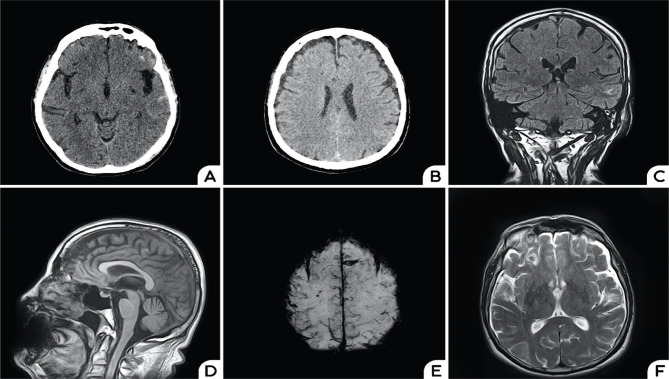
Axial native CT scan showing subarachnoid hemorrhage located in the frontal lobes bilaterally and in the left temporal lobe (A); Follow-up CT scan performed 48h later showing bilateral hygromas (B); Coronal FLAIR showing superior sagittal sinus engorgement, dilated cortical veins, hemorrhage in the left temporal lobe (C); Sagittal T1-weighted imaging showing sunken splenius (D); Axial SWI showing superficial siderosis (E); Axial T2-weighted imaging showing hygromas and hemorrhage in the right frontal lobe (F).
